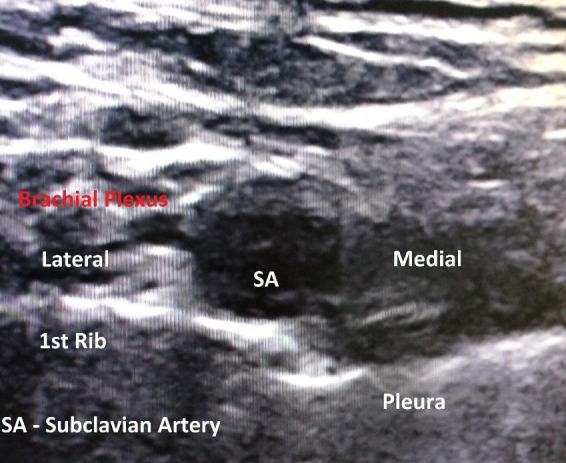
Ultrasound image 24 hours after block.
